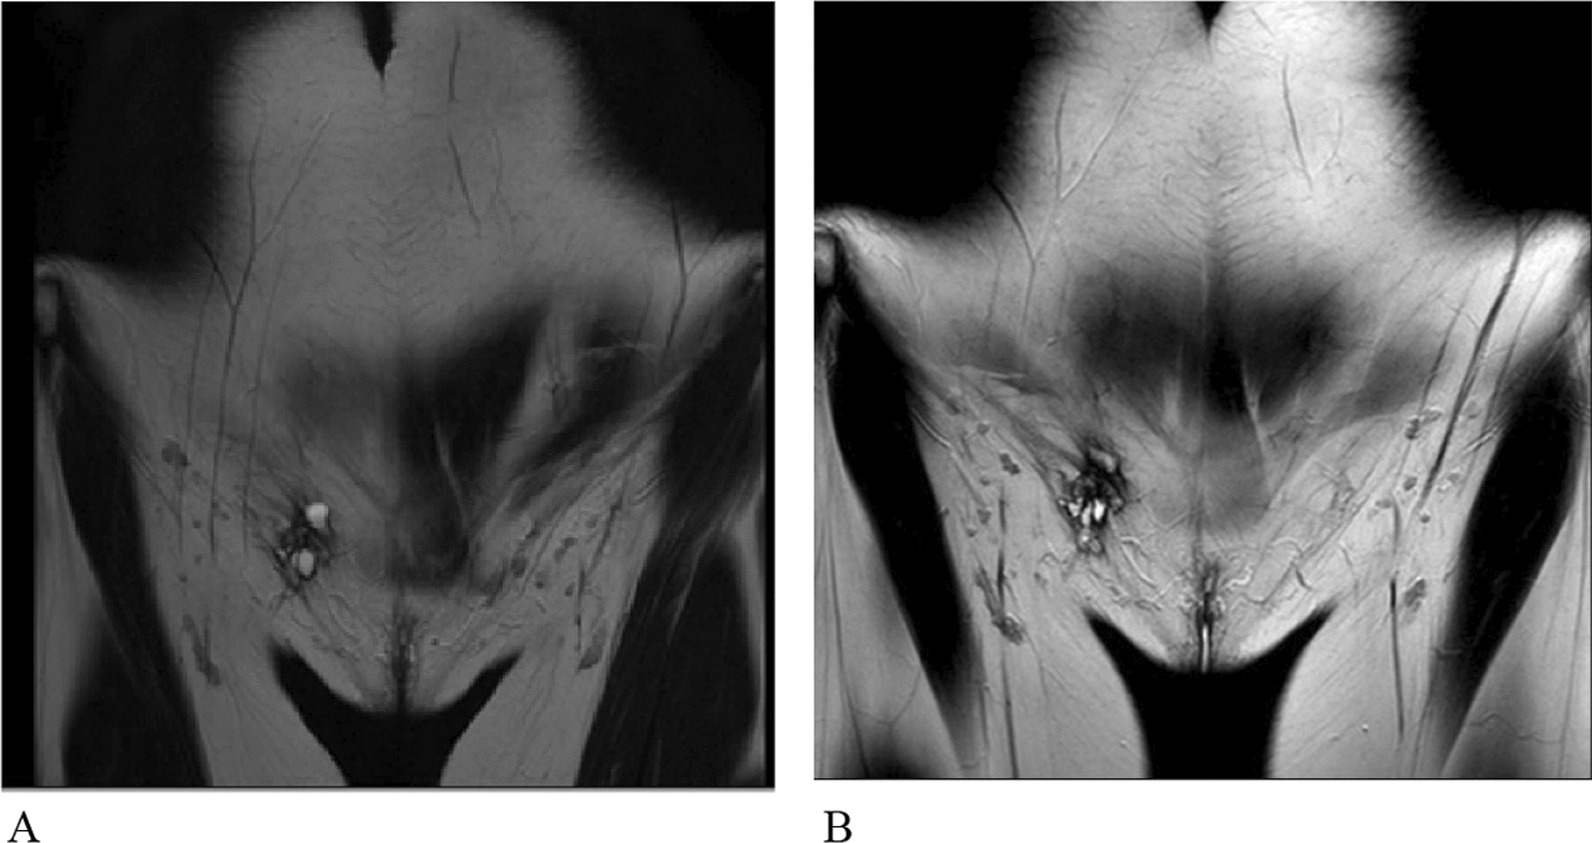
Comparison of inguinal endometriosis size in magnetic resonance imaging. A first magnetic resonance imaging (35 × 9 mm); B second magnetic resonance imaging 9 months later (61 × 60 mm)
